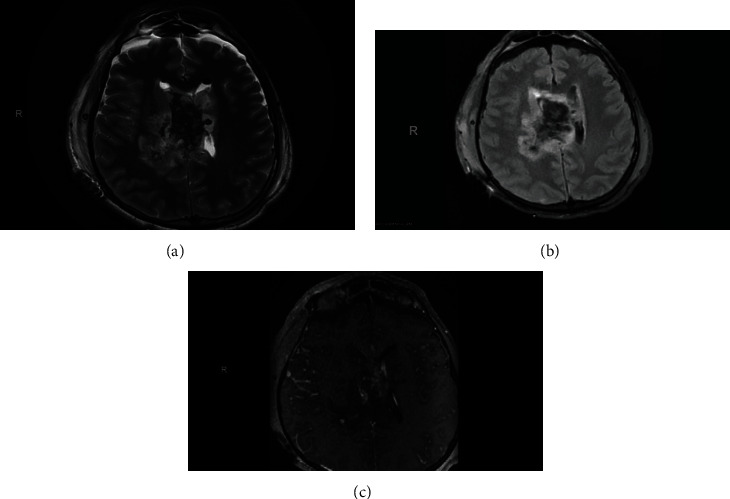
Brain MRI axial view T2 T1 postop.
